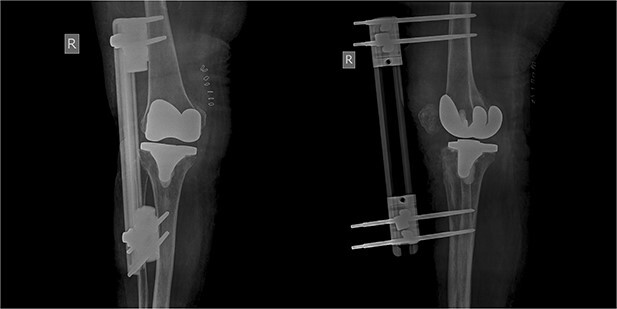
X-rays 1-month after external fixation.
